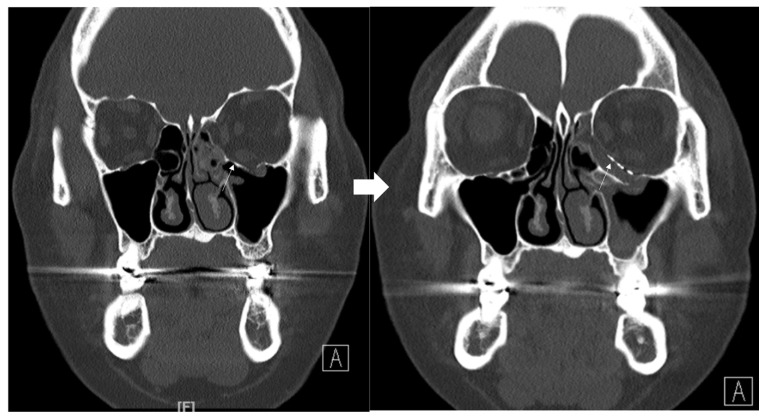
Case 4. Preoperative facial CT scan (coronal view) of the 51-year-old female patient with left inferomedial orbital wall fracture (white arrow) and follow-up facial CT finding (coronal and axial view) of the same patient.
